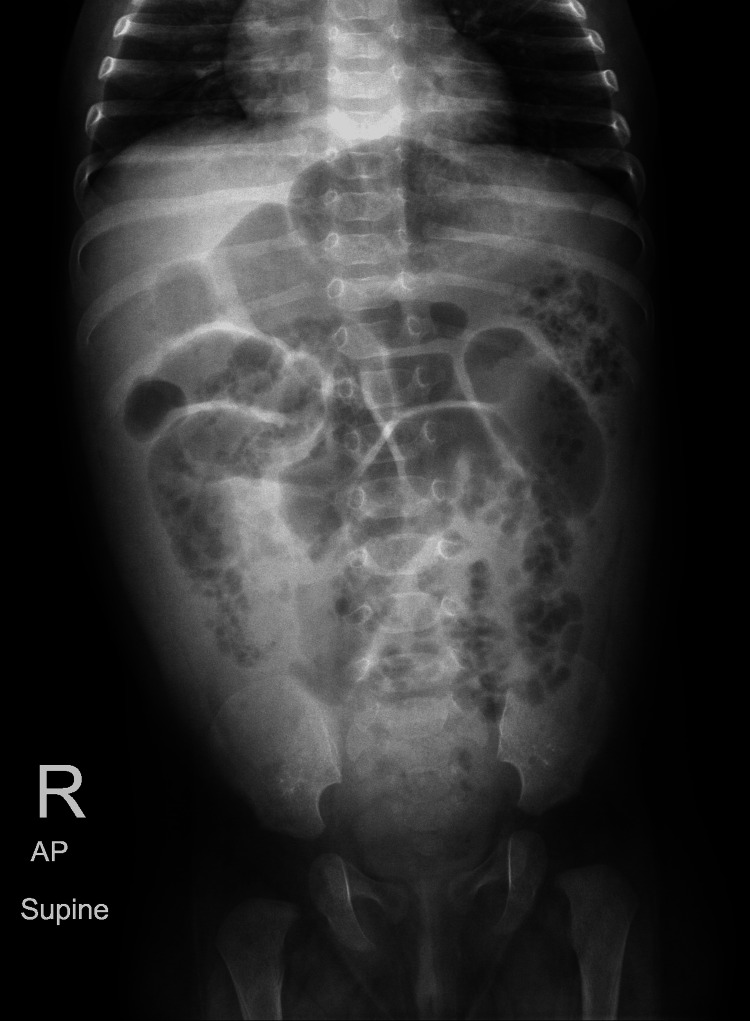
Abdominal X-ray. Moderate gaseous distension of the stomach and the bowel loops are noted in the center of the abdomen with a “soap-bubble” appearance of the nondistended bowel loops in the periphery with rectal air.
